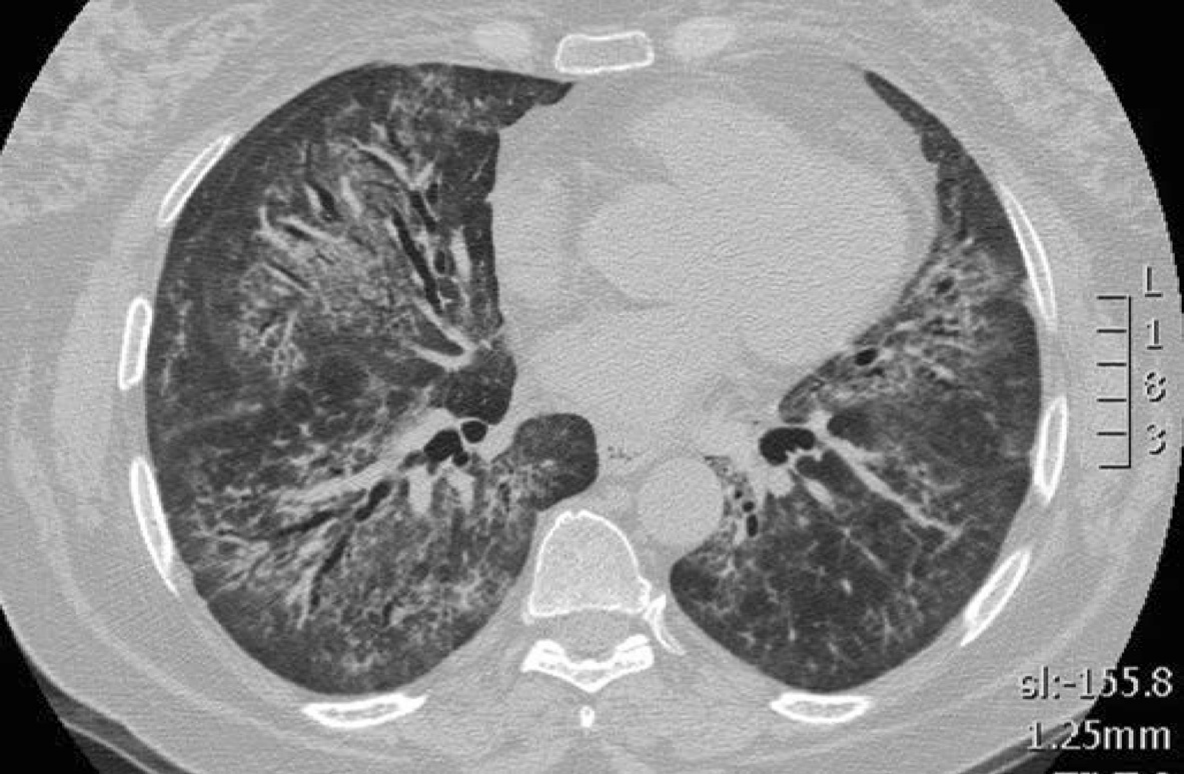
CT scan of a 52 years old lady, affected by idiopathic NSIP. Bilateral, peribronchovascular ground glass attenuation, due to intralobular fibrotic changes. Traction bronchiectasis are present bilaterally surrounded by ground glass,“fibrotic” attenuation, mainly in the right middle lobe and in both lower lobes. No honeycombing is present. A relative subpleural sparing is also visible
